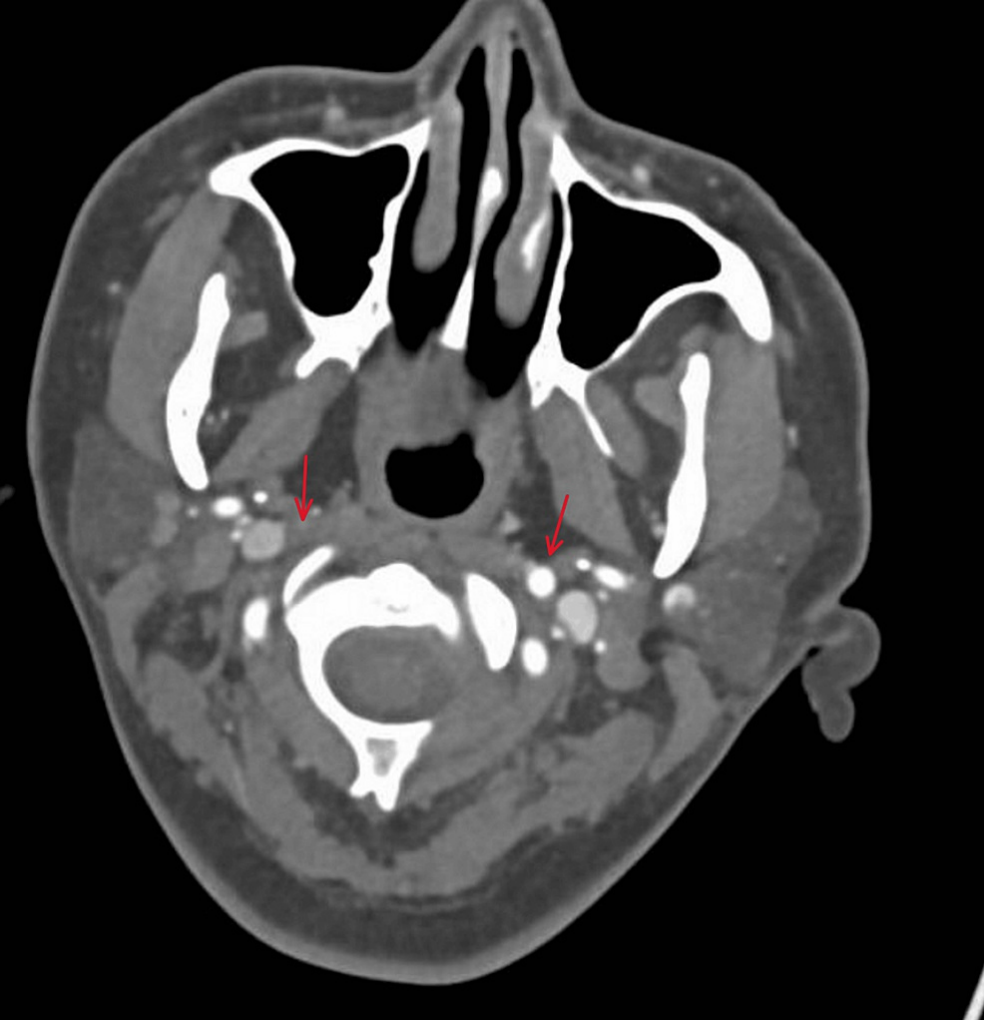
CTA of the head showing occlusion of RICA. CTA = computed tomography angiography, RICA = right internal carotid artery
